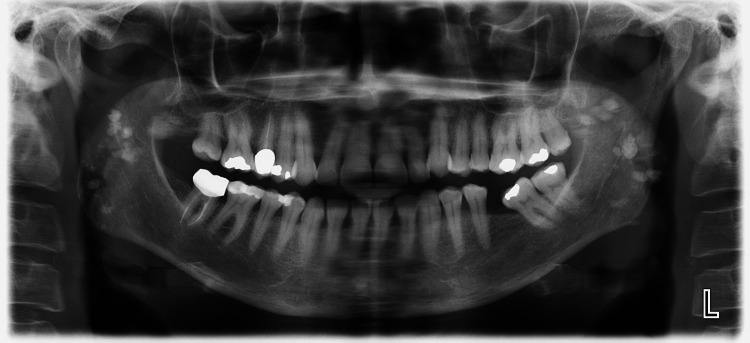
Panoramic radiograph showing calcifications in the sternocleidomastoid muscle.
